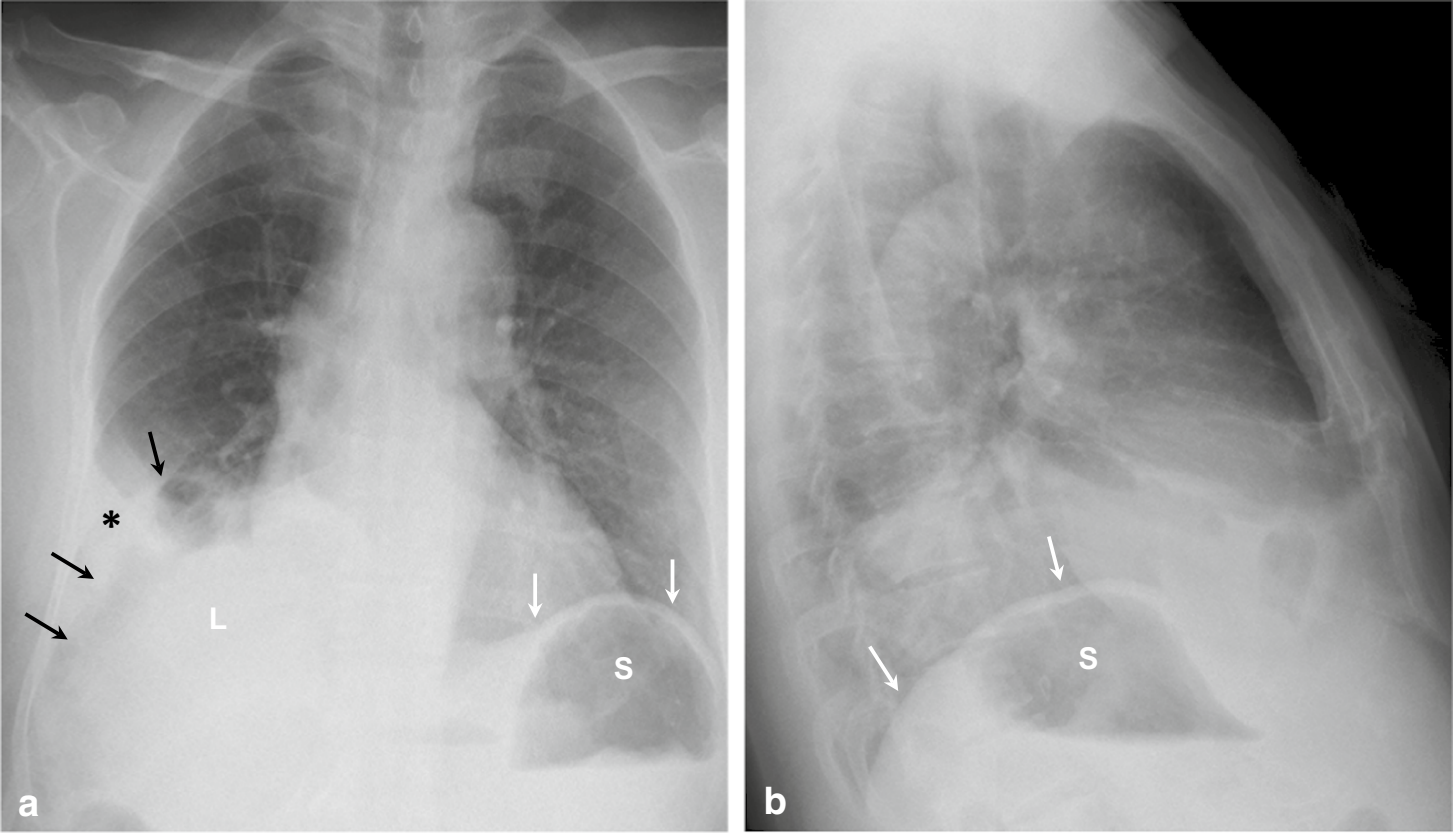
Plain radiograph of the chest: frontal (a) and lateral view (b) demonstrate non-visualization of left dome of the diaphragm on both views. Note the normal left hemidiaphragm (white arrows in a and b) with air bubbles within the stomach (S) below. A bowel loop (black arrows in a) is seen extending above the liver shadow (L in a) at the lower chest. A small pleural effusion (*) is also present
